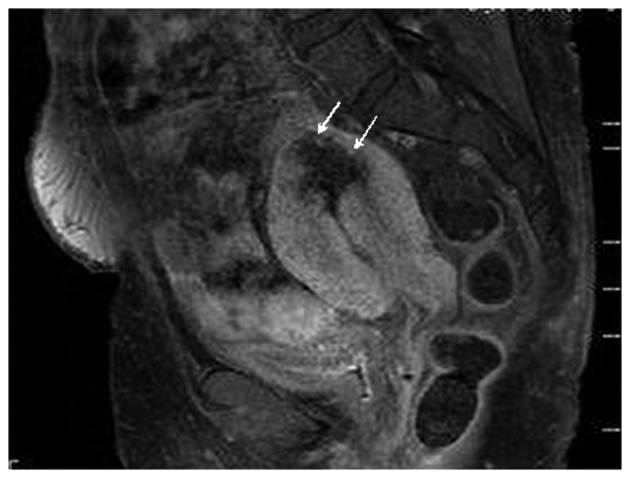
Magnetic resonance imaging showing a relatively well-circumscribed tumor in the fundus of the uterine corpus (arrows).
